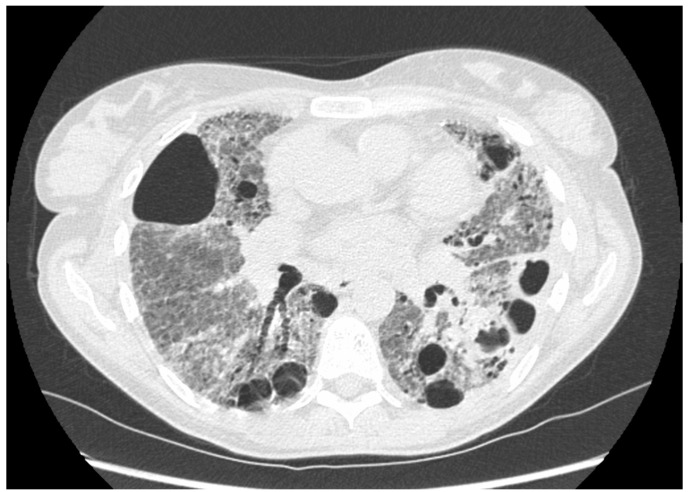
Chest HRCT, axial planes: CT scan shows GGO, subpleural nodules or consolidations, bronchovascular bundles thickening, interlobular septal thickening, thin-walled cystic airspaces with perivascular distribution, and architectural distortion.
